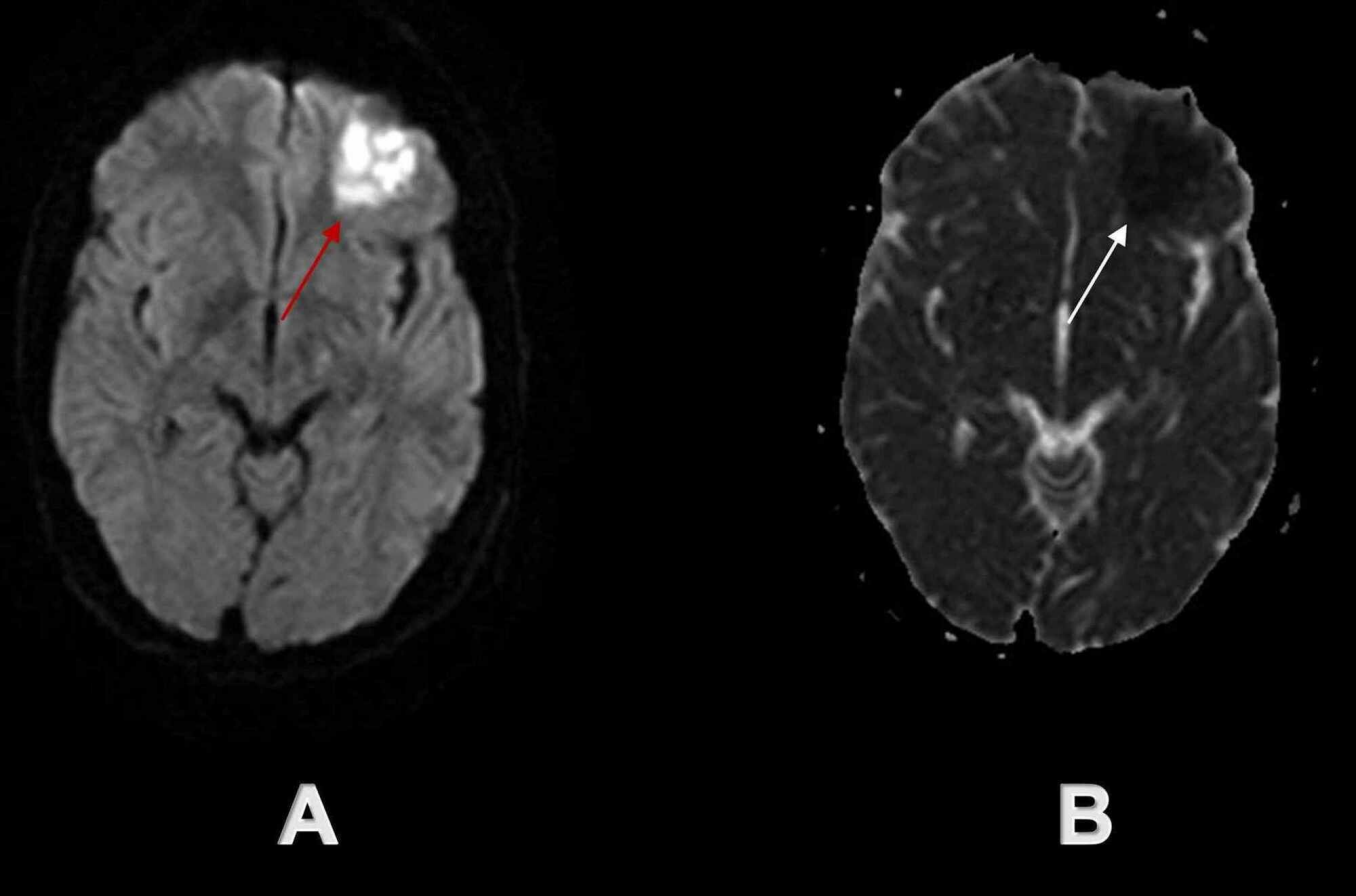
Initial brain MRI demonstrated acute ischemic infarct in the left frontal lobe The red arrow on axial DW sequence (A) and white arrow on axial ADC sequence (B) demonstrate acute ischemic infarct in the left frontal lobe. DW - diffusion-weighted; ADC - apparent-diffusion-coefficient
